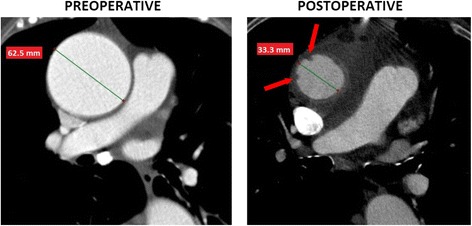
A comparison of preoperative and postoperative angio-CT images of a patient whose aorta was decreased by 47 %. Red arrows point at the sites of plication of the aortic wall
